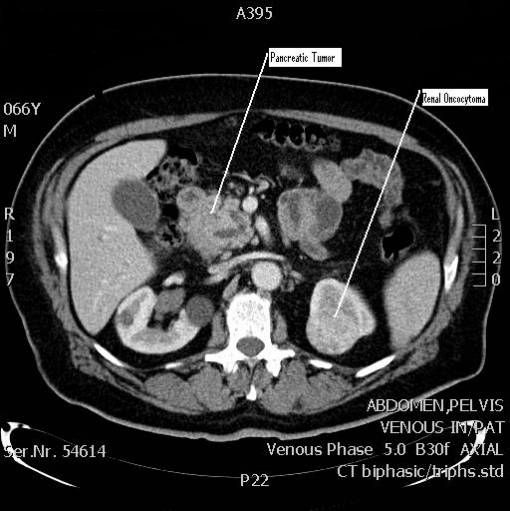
CT scan of the abdomen showing the pancreatic tumor and the left renal oncocytoma.
